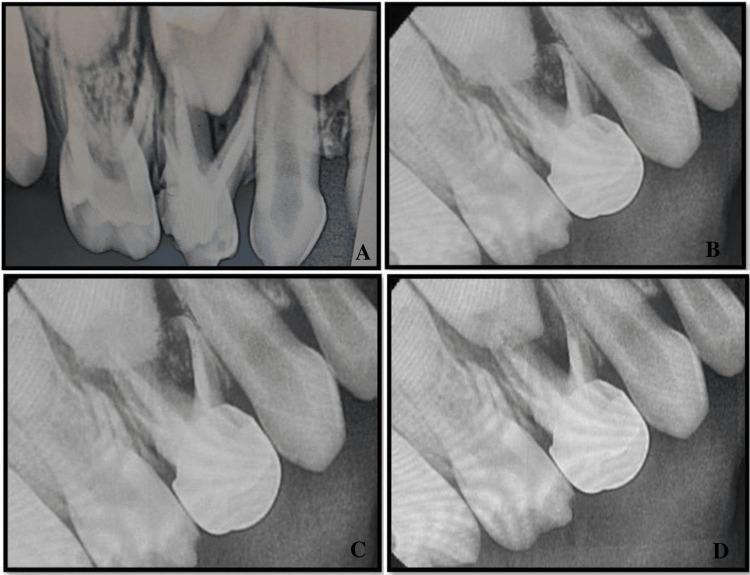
Radiographic assessment (A) Immediate postoperative radiograph. (B) Postoperative radiograph at three months. (C) Postoperative radiograph at six months. (D) Postoperative radiograph at nine months.
